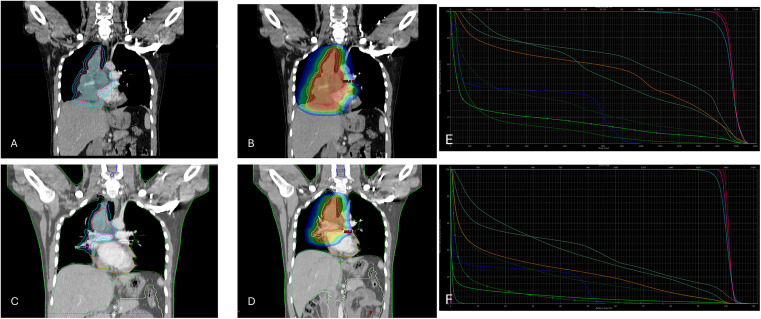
Dose distributions and dose volume histograms of the staged adaptive thoracic radiotherapy strategy. (A) Initial planning CT with target and selected organ-at-risk contours. (B) Initial IMRT dose distribution. (C) Repeat planning CT after tumor regression and right lung re-expansion with adaptive target and organ-at-risk contours. (D) Adaptive VMAT dose distribution. (E) Dose volume histogram of the initial plan. (F) Dose–volume histogram of the adaptive VMAT plan.
